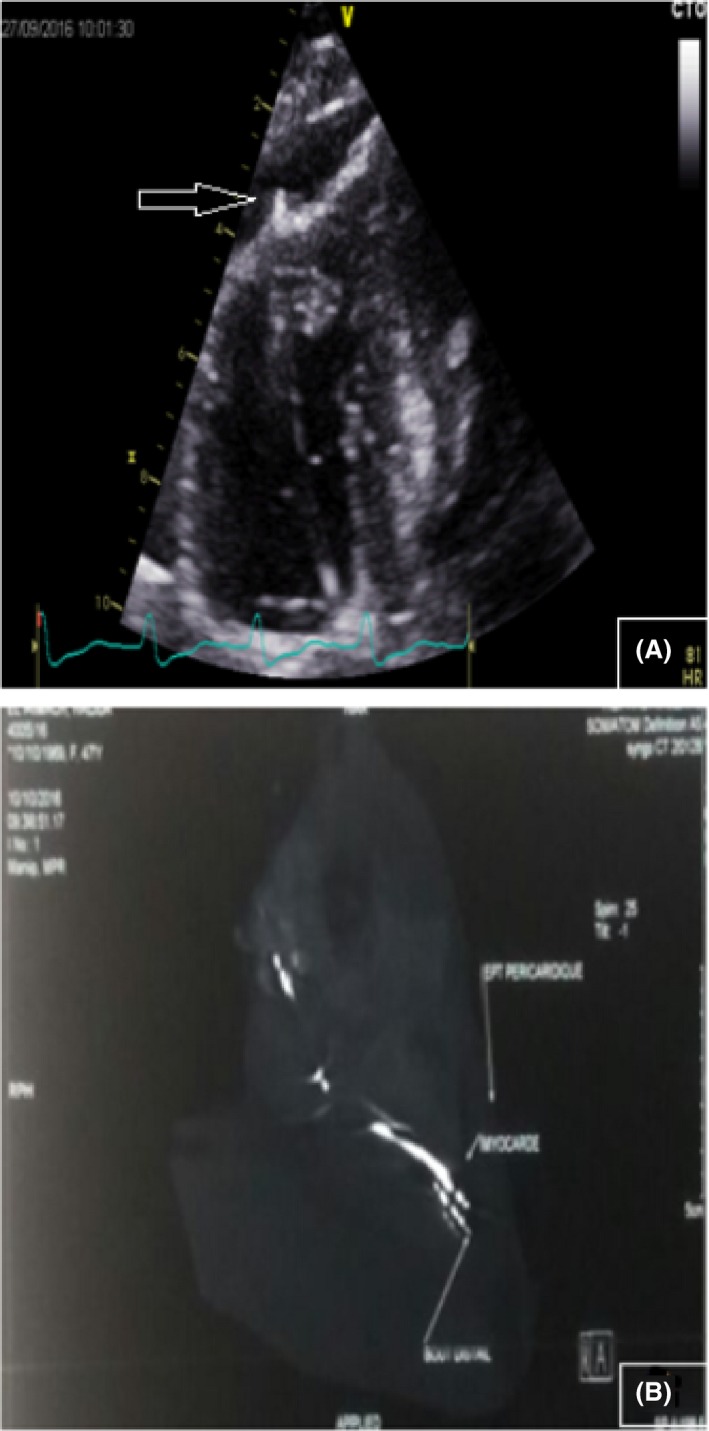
(A) Zoom of right ventricle in the apical four‐chamber view: lead tip visualized in the pericardial space, confirming perforation (arrow). (B) Chest computed tomography showing a myocardial perforation by the pacing lead (arrow). Note the associated pericardial effusion (arrow).
